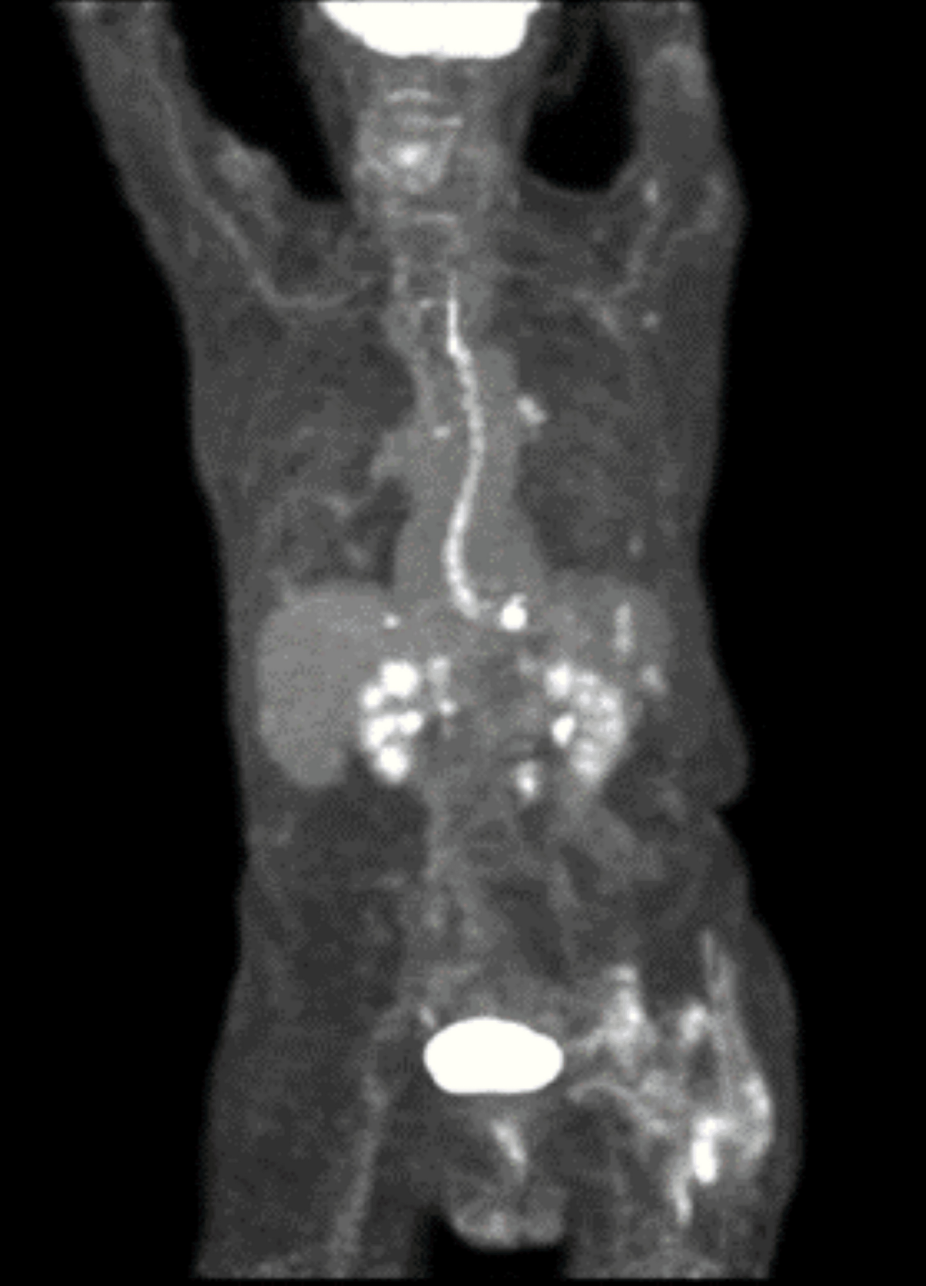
PET/CT between months 14-15 after diagnosis Full-body image demonstrating multiple areas of high FDG uptake, consistent with widespread lymphoproliferative disease. CT, computed tomography; PET, positron emission tomography; FDG, fluorodeoxyglucose
